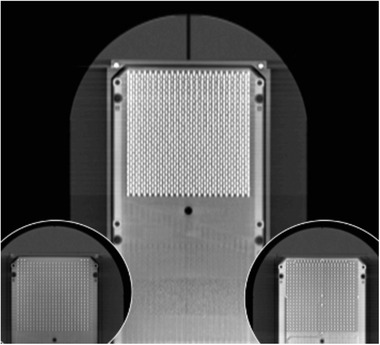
CT coronal reconstructed image that shows the diodes distribution in two PCBs. The small pictures below show the two complementary printed circuit boards (PCBs) that are overlapped and aligned in such a way that the active zone of the pn‐junction is on the same plane forming a single measurement plane
